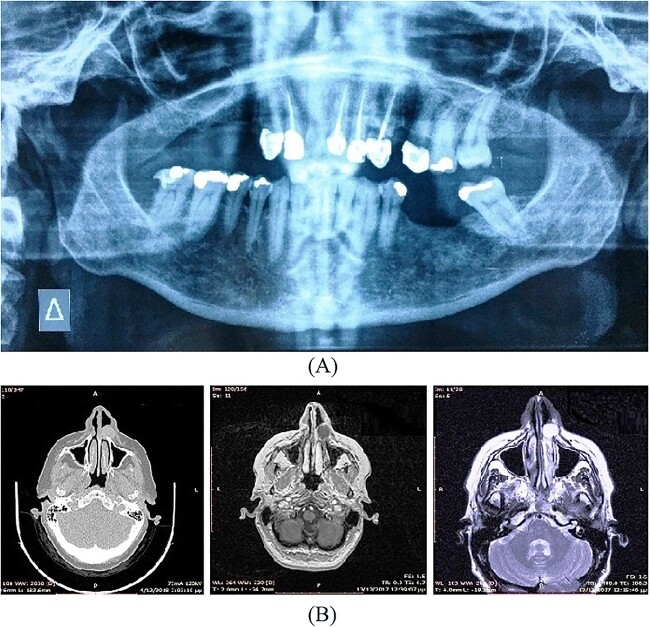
(A) Orthopantomogram (B) CT, MRI image of the lesion.
